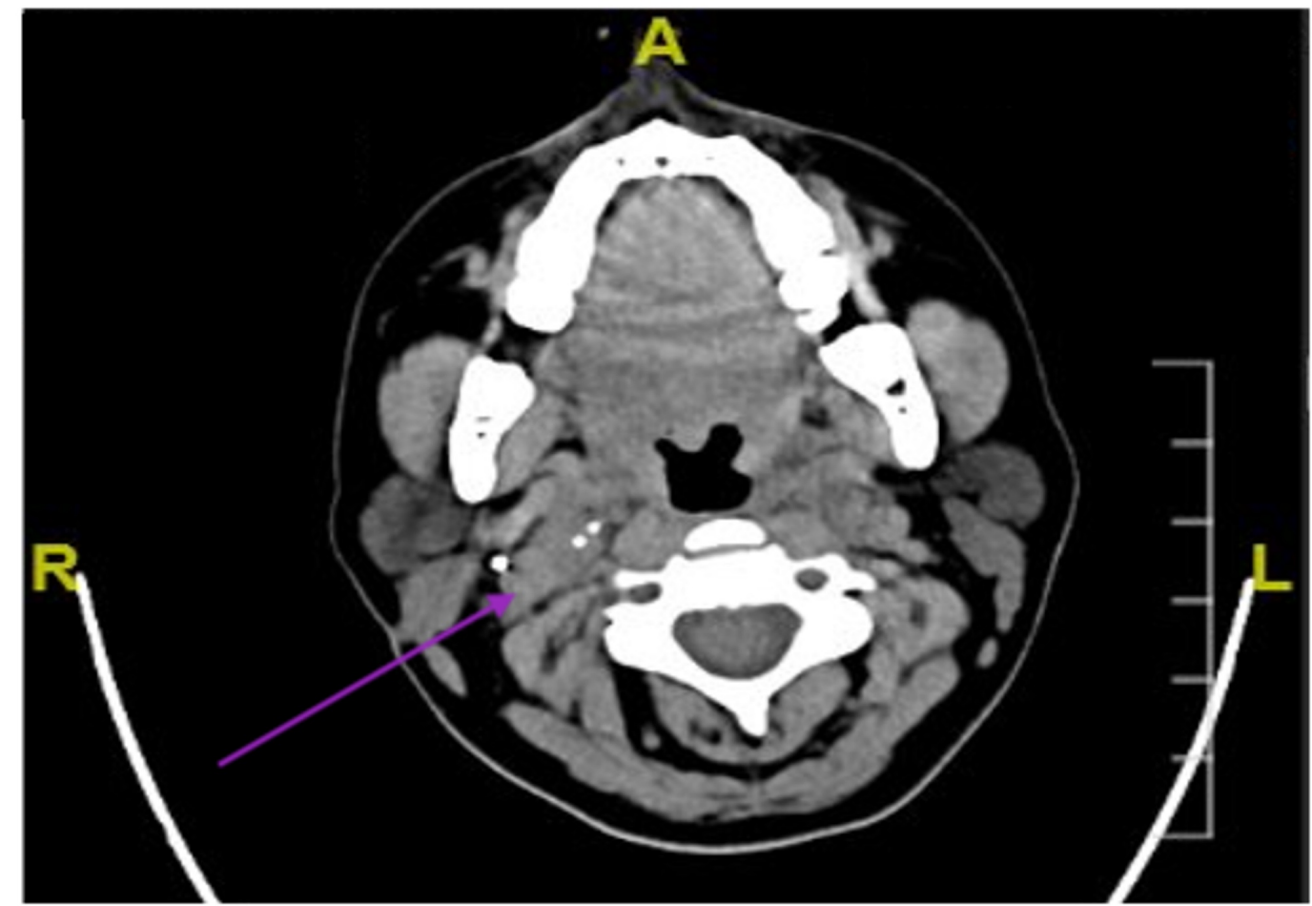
Post-surgical resection computed tomography
